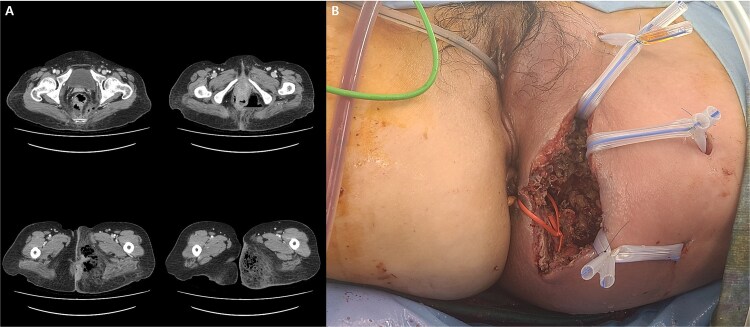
(A) Abscess formation with emphysema in the left perineum and buttock on abdominal pelvic CT scan. (B) Postoperative images after incision and drainage.
